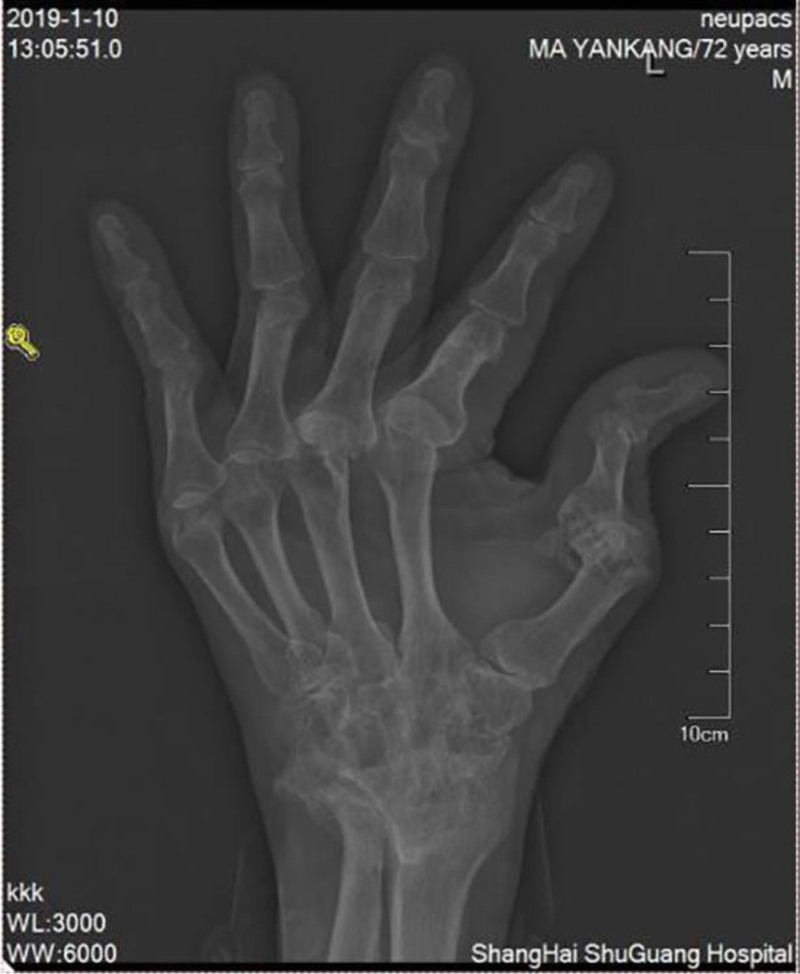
Plain radiographs of limb joints showed MCP2 bone erosion in the left hand.
